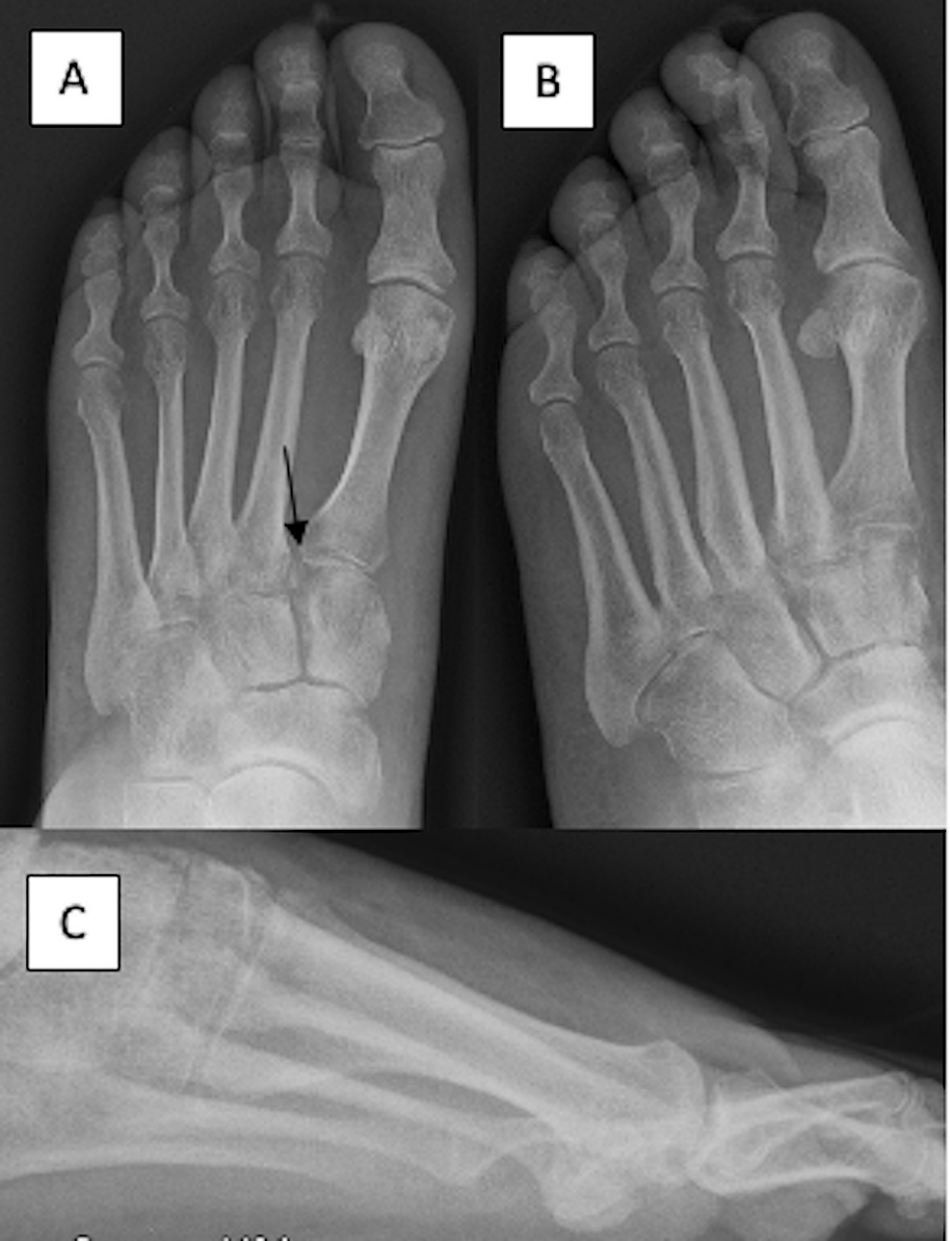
Foot radiographs. Radiographs of the foot seven weeks after the index injury demonstrating an avulsion fracture from the base of the second metatarsal consistent with Lisfranc injury.
